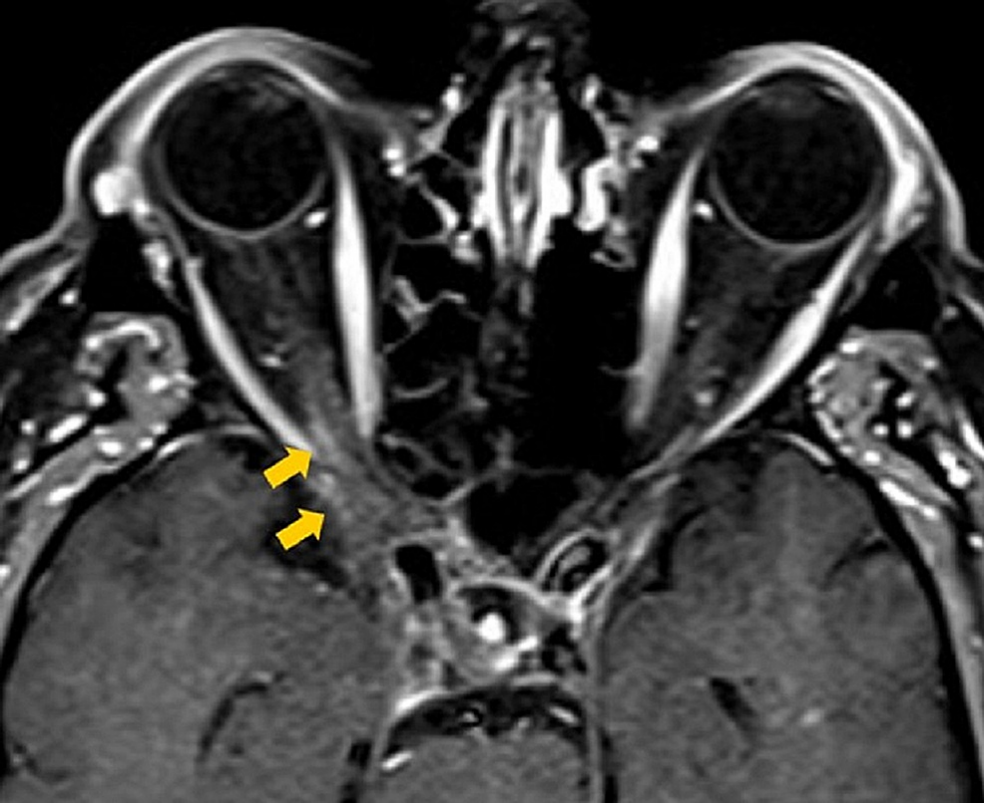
Three-month follow-up axial contrast-enhanced T1-weighted MRI The image is showing markedly decreased inflammation of the right posterior orbital apex and right cavernous sinus (yellow arrows) after treatment with prednisone 60 mg.
